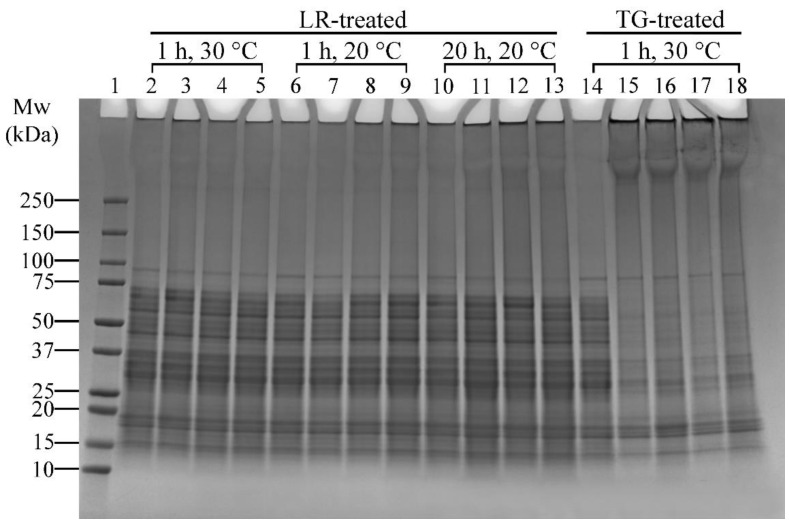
SDS-PAGE profile showing the effects of varying laccase (LR) (under the addition of ferulic acid—FeA), transglutaminase (TG) concentrations, and incubation temperatures on lupin and soy (LS) protein mixtures. Lane 1: Protein marker; Lane 2: LS-LR-285U-1H-30C; Lanes 3–5: LS-LR-71.25U/142.5U/285U-FeA-1H-30C; Lane 6: LS-LR-285U-1H-20C; Lanes 7–9: LS-LR-71.25U/142.5U/285U-FeA-1H-20C; Lane 10: LS-LR-285U-20H-20C; Lanes 11–13: LS-LR-71.25U/142.5U/285U-FeA-20H-20C; Lane 14: LS-CT-1H-30C; Lanes 15–18: LS-TG-1.25U/2.5U/5U/10U-1H-30C. CT—Control (non-enzyme-treated); U—Enzyme units (U/100 mg protein); H—Treatment time (h); C—Incubation temperature (°C). *Summary:*
